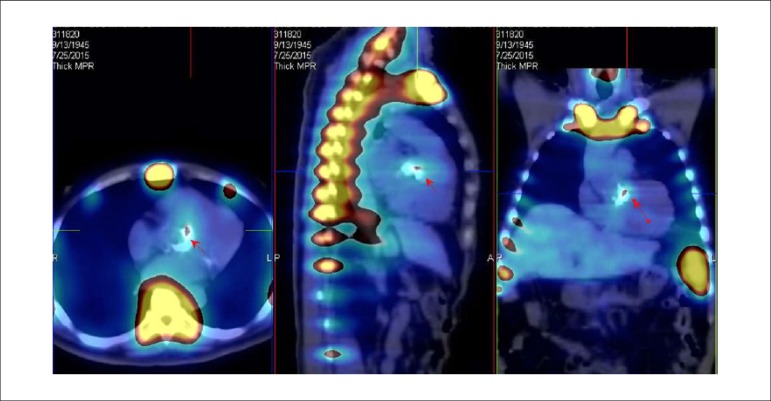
Images from scintigraphy with labeledleukocytes with SPECT/CT demonstrate anomalous accumulation in the area of the percutaneously implanted aortic valve (arrows). Transesophageal echocardiogram was inconclusive, and blood culture was positive for Staphylococcus aureus. The patient was diagnosed with prosthetic valve infective endocarditis.
